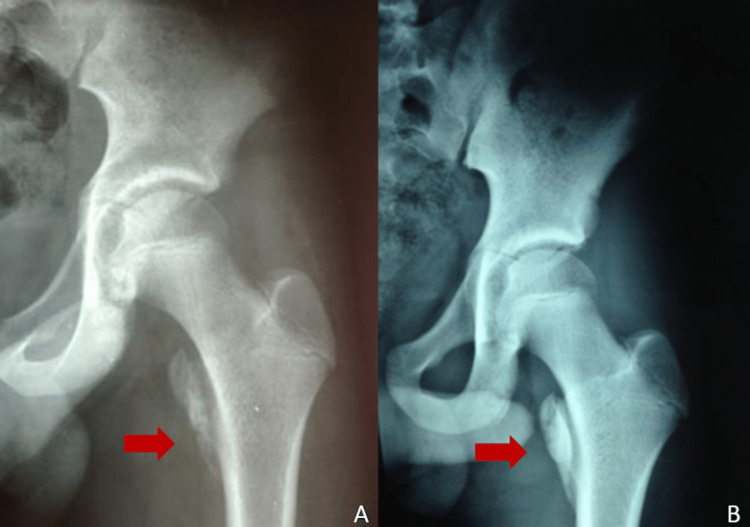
AP radiographs of the left hip at two (A) and six (B) months after the injury, indicating the callus formation in the posttraumatic period (red arrows).
